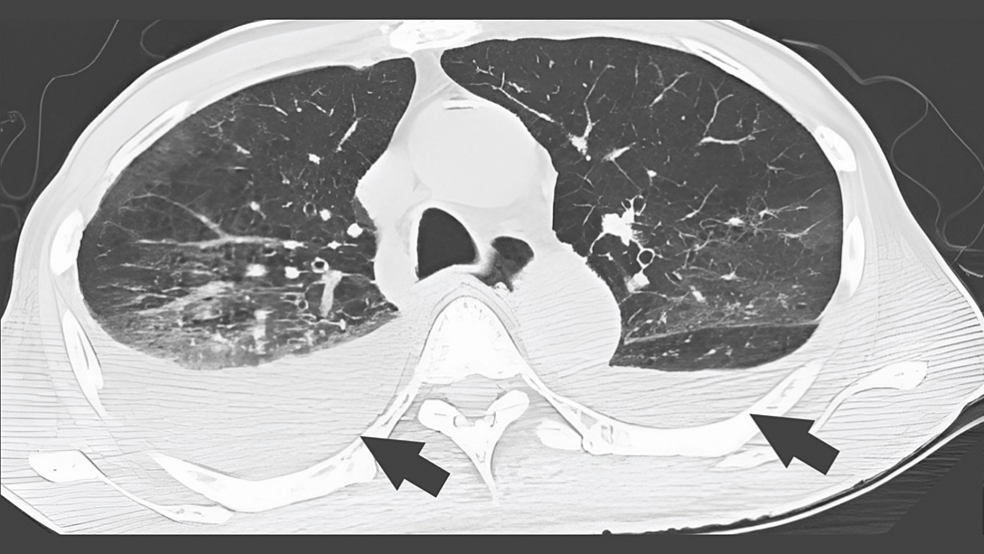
Chest computed tomography showing the bilateral lung frosted shadows and pleural effusions.
